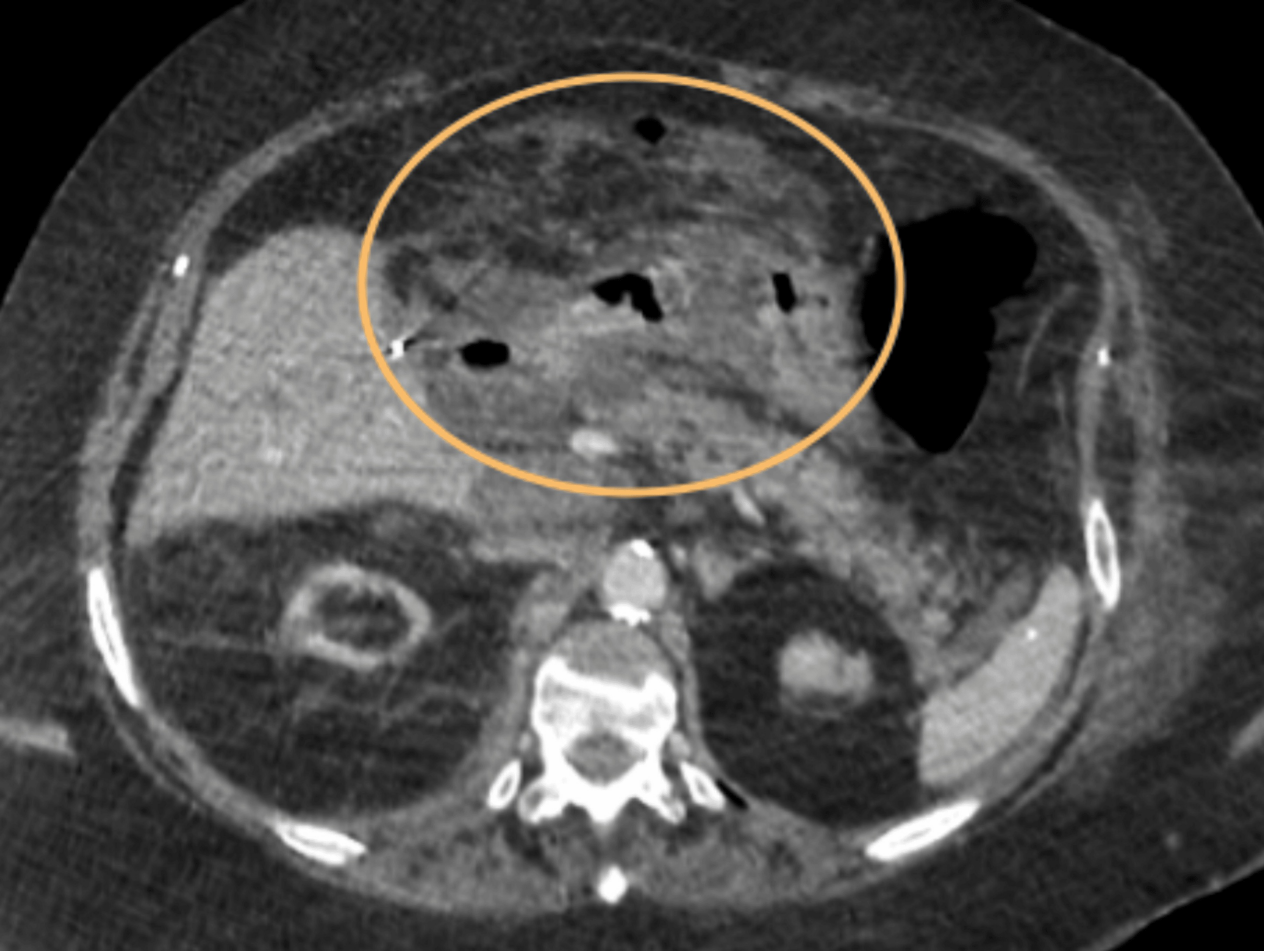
Computed tomography (CT) scan of the abdomen and pelvis with contrast, showing sequelae of acute pancreatitis, with an acute peripancreatic fluid collection in the pancreaticoduodenal groove, measuring approximately 2.3 by 2.9 cm (labeled by the orange circle)
